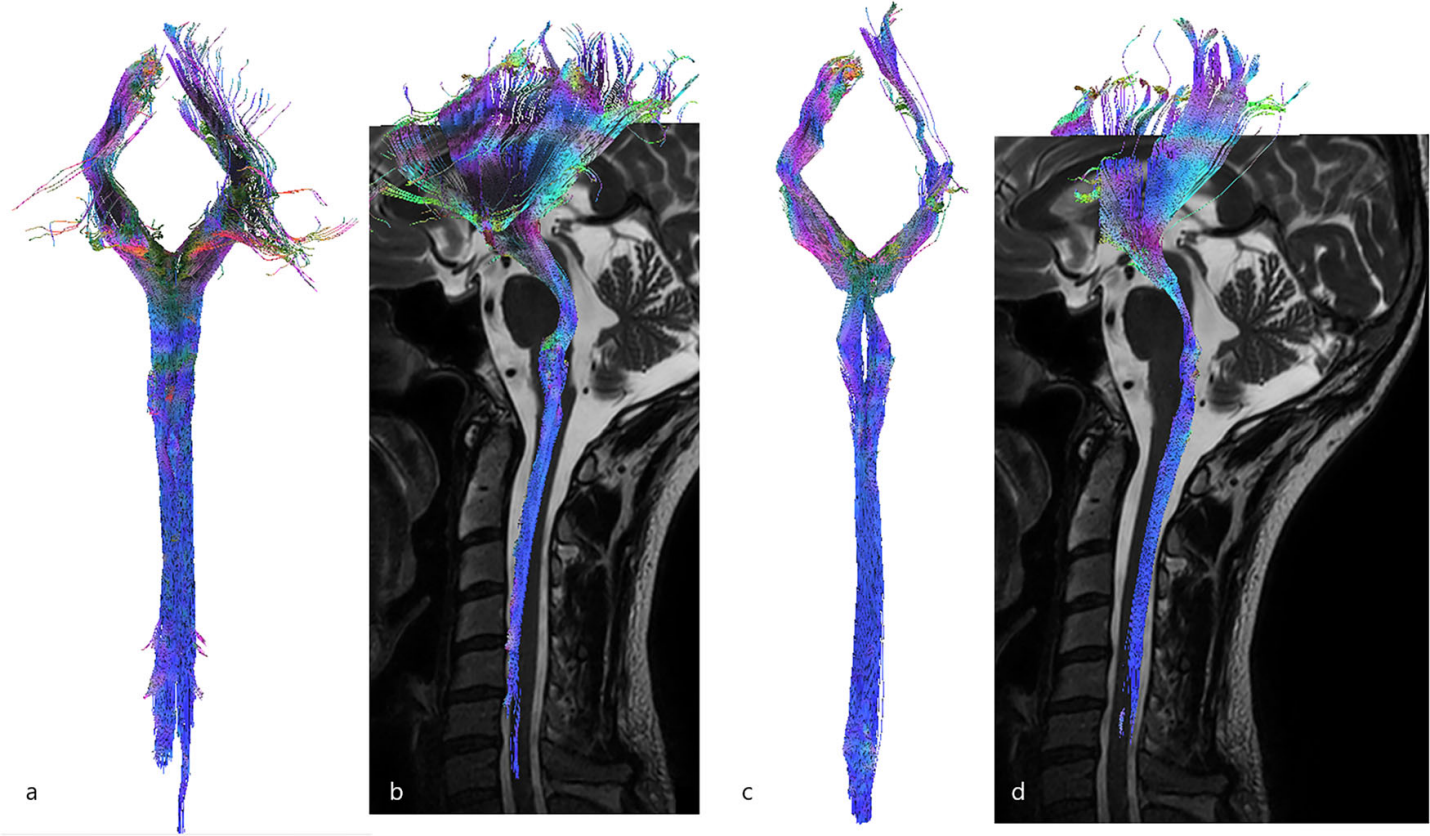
3D-tractography rendering of the sensitive pathways. a Coronal view of the spinothalamic tract. b Sagittal view of the spinothalamic tract merged on sagittal T2-weighted images. c Coronal view of the dorsal columns. d Sagittal view of the dorsal columns tracts merged on sagittal T2-weighted images
